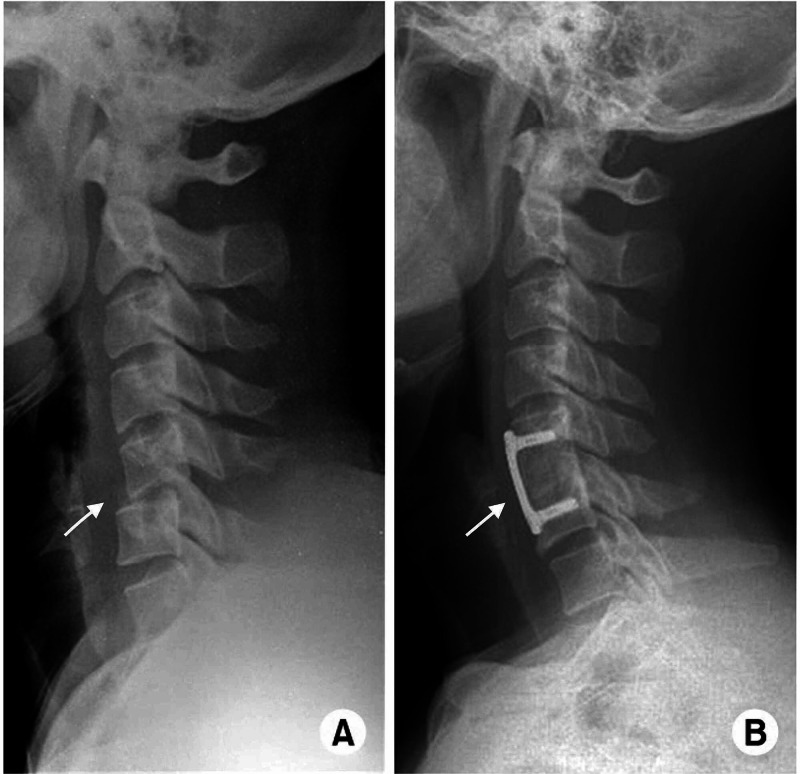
C5-6 unilateral facet joint dislocation. Lateral radiographs of the cervical spine demonstrating (A) unilateral facet joint dislocation at the C5-C6 level and (B) two years after operation (ACDF) showing well reduction and fixation state ACDF, anterior cervical decompression and fusion Image courtesy: ResearchGate. Available from https://www.researchgate.net/figure/C5-6-unilateral-facet-joint-dislocation-Lateral-radiographs-A-of-the-cervical-spine_fig14_274058208 (accessed November 25, 2019), via license: Creative Commons Attribution-NonCommercial 3.0 Unported.
